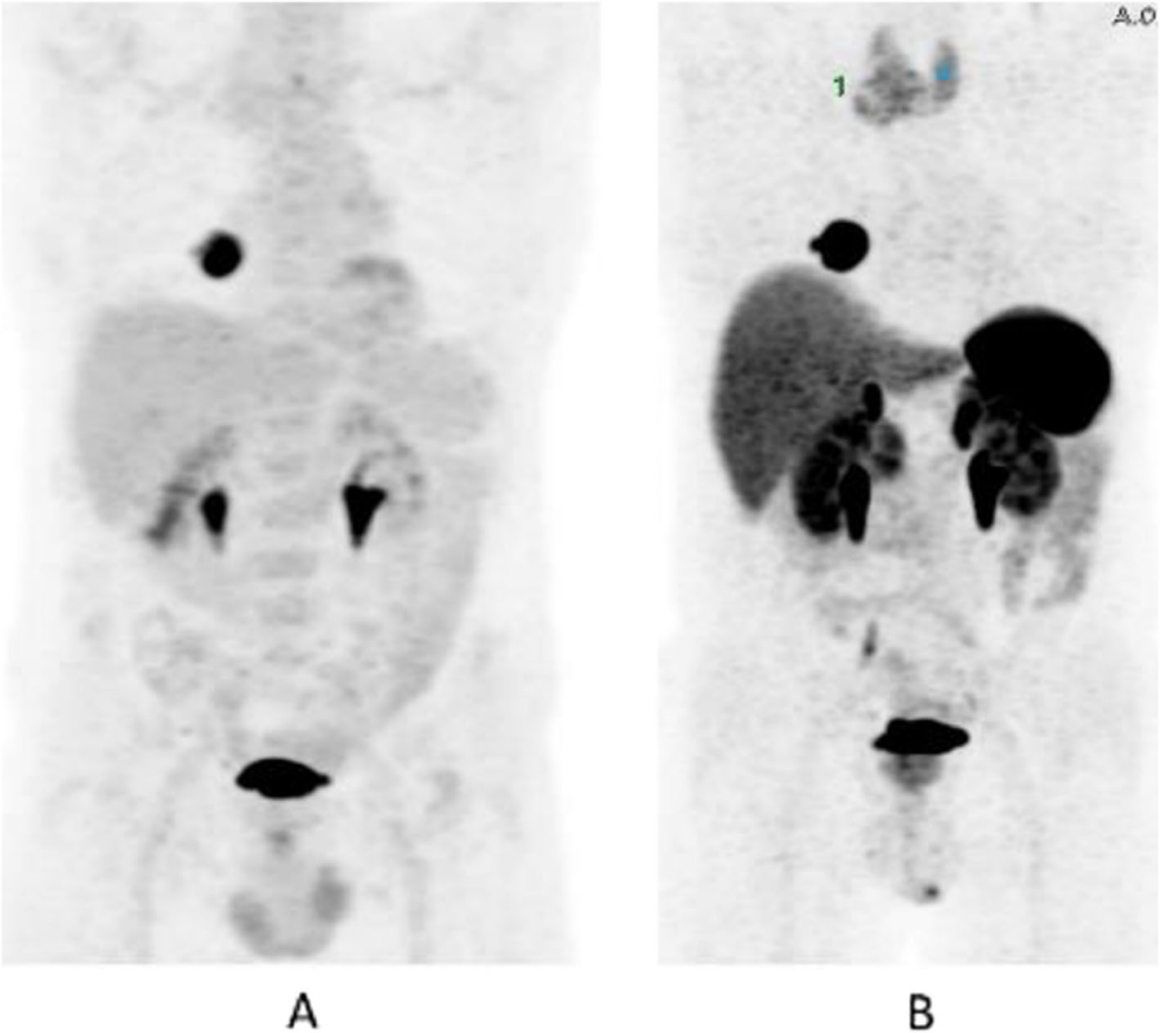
^68^Ga-DOTA peptide, staging of NET lung primary, comparison with FDG. *Clinical history*: 65 y.o. man. Staging of a lung mass with FDG PET and biopsy indicated a moderately differentiated NET (grade 2, Ki67 8%). Consequently, the patient underwent a second PET using ^68^Ga-DOTANOC to stage the disease more accurately. *PET/CT findings*: increased focal uptake in FDG PET in the right lung, without any other findings (a MIP). ^68^Ga-DOTANOC showed intense uptake in the lung and in the thyroid due to a known De Quervain thyroiditis (b MIP)
